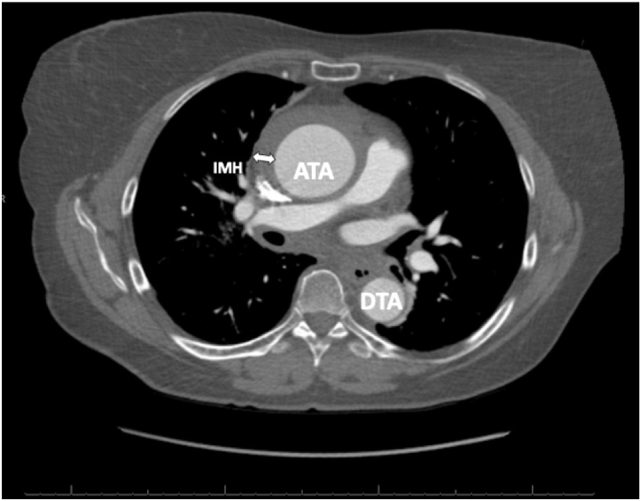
Contrast-enhanced CT scan, axial display at the proximal ascending thoracic aorta, demonstrates the IMH (*arrow*) as an unenhanced, crescentic, low-attenuation (relative to the aortic lumen) region of the aortic wall thickening with no evidence of an aortic dissection or intimal flap at this level. *ATA*, Ascending thoracic aorta; *DTA*, descending thoracic aorta.
